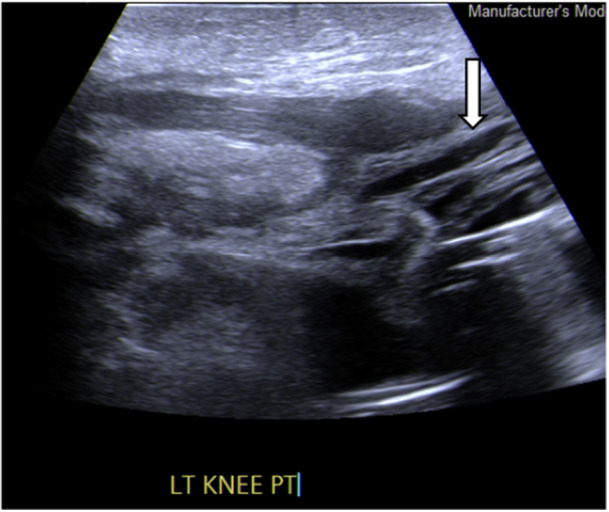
Long‐axis ultrasound image demonstrating ruptured patellar tendon (PT) from its attachment over the tibial tuberosity with an organized haematoma (marked by the arrow) at the retracted tendon site anterior to the tibial tuberosity in the deep infrapatellar bursal region.
